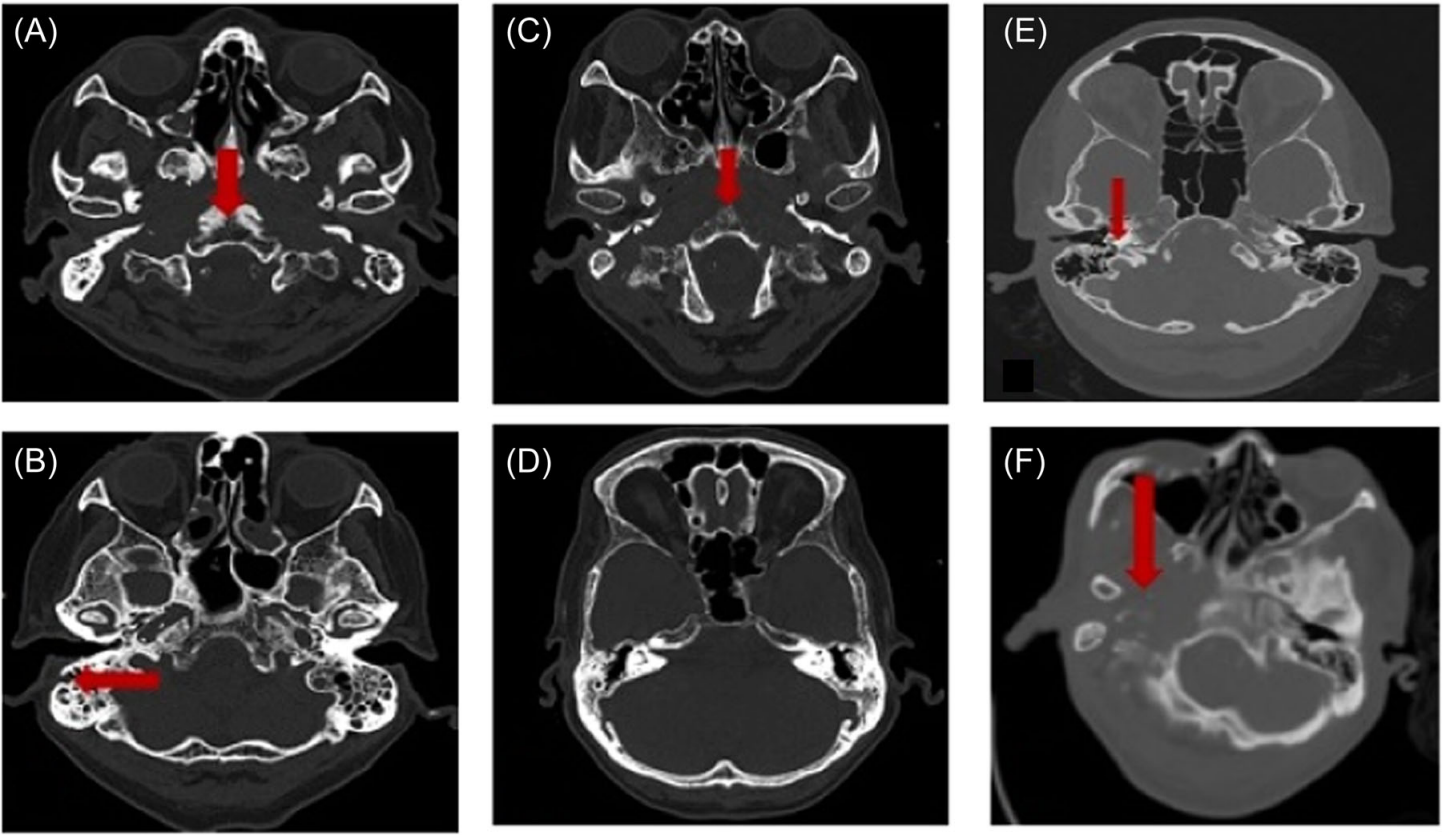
Figures (A–F) show multiple axial cuts of a computed tomography scan imaging of skull base osteomyelitis. Red arrow indicates the site of erosion.
